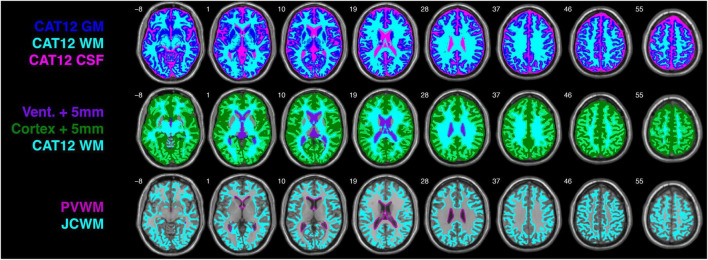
CAT12 gray matter (GM), white matter (WM), and cerebrospinal fluid (CSF) tissue segmentations from the single-subject T1-weighted ICBM152 image (top row), ventricle and cortical masks following 5 mm 3D dilation with the CAT12 WM mask (second row), and periventricular WM (PVWM) and juxtacortical WM (JCWM) masks. For the purposes of this investigation, PVWM is defined as WM within 5 mm of the ventricles, and JCWM is defined as WM within 5 mm of the cortex.
